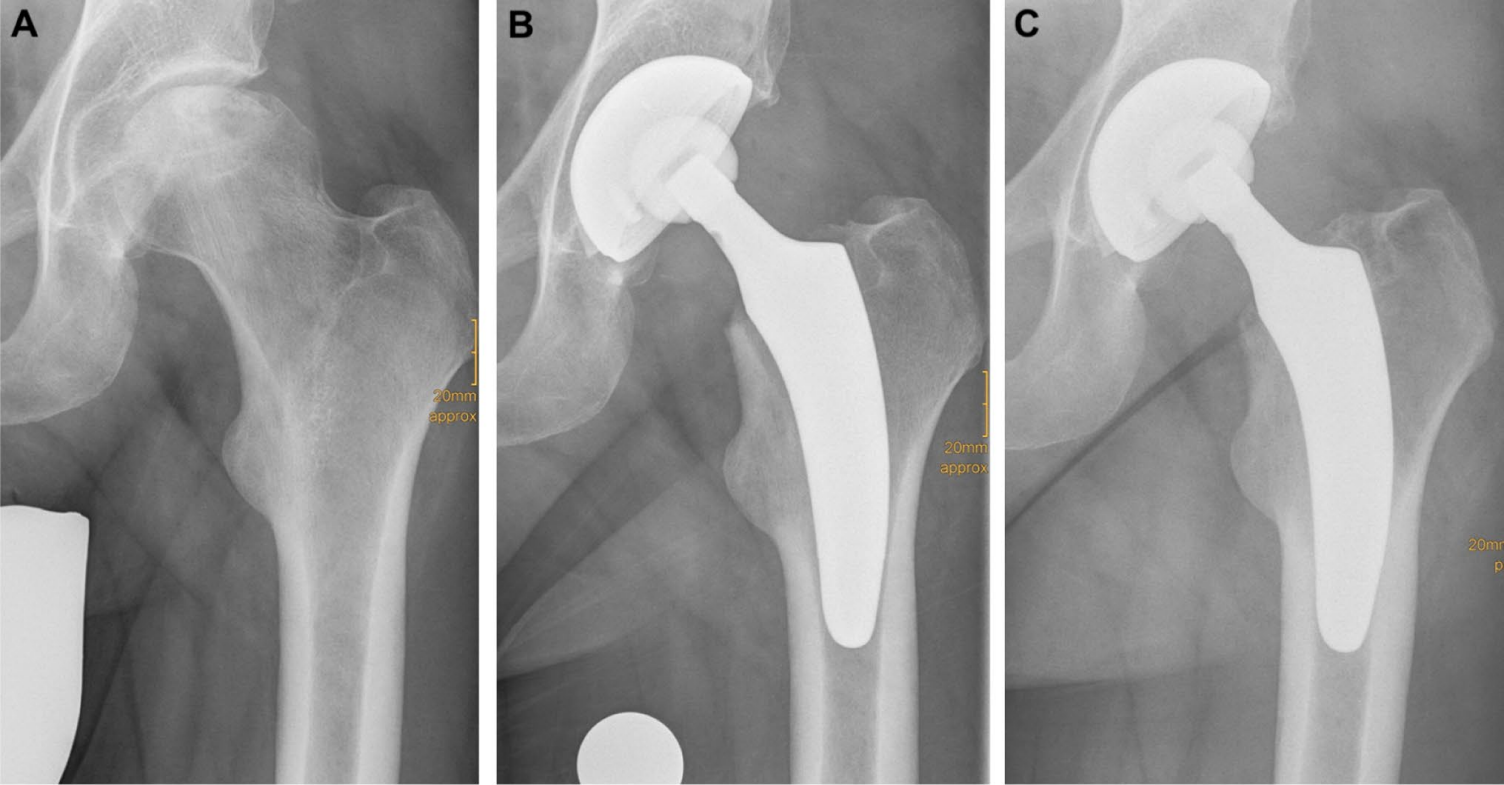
Radiographs of a 49-year-old male patient (weight: 110; height: 186 cm) with ONFH. a Preoperative (ARCO stage IV and Dorr type A); b postoperative; c mid-term follow-up (axial migration of 5 mm, without signs of stem loosening)
